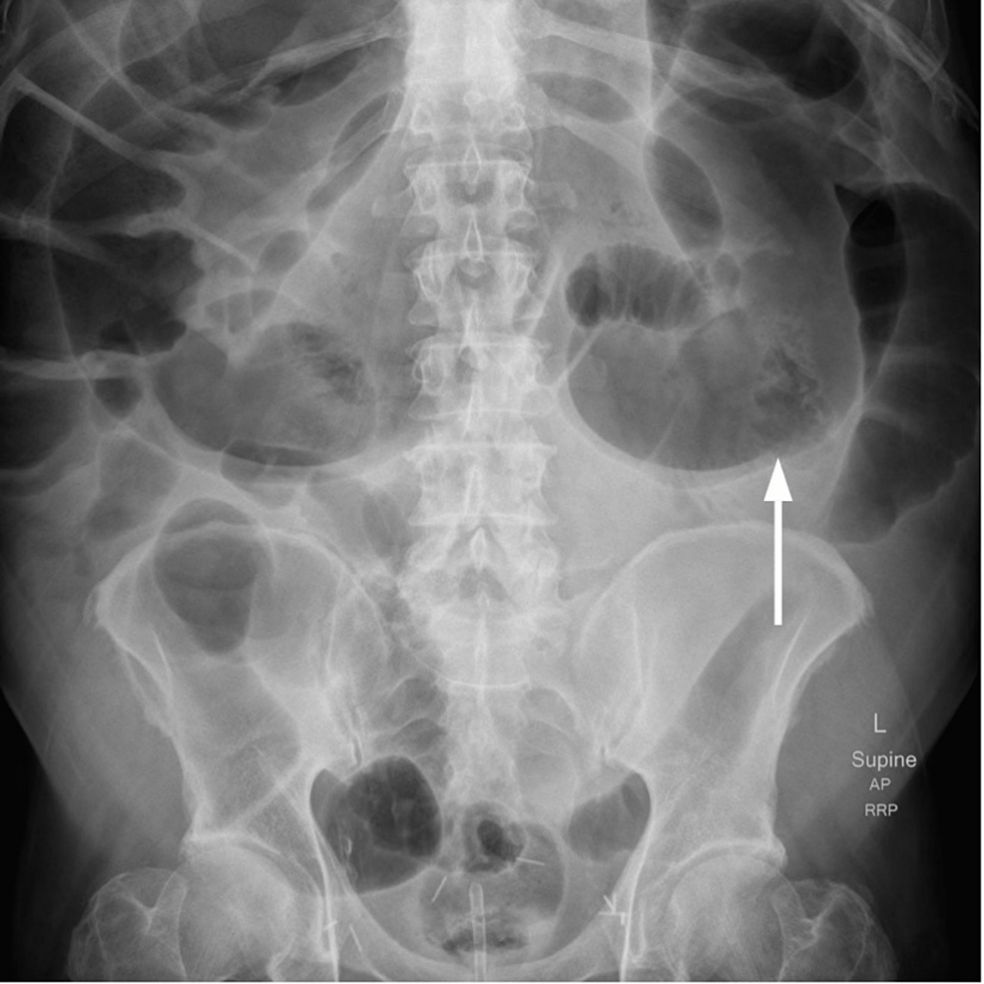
Abdominal X-ray The arrow demonstrates colon distention
